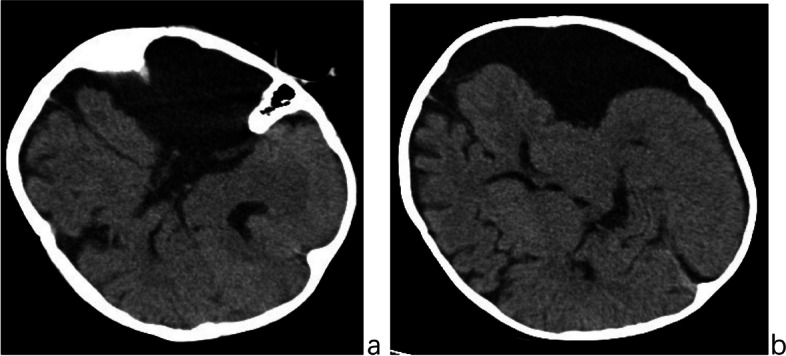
a and b Cranial CT scan showing a large left frontotemporal arachnoid cyst (a) and associated subdural effusion in the left frontotemporal parietal region (b)
